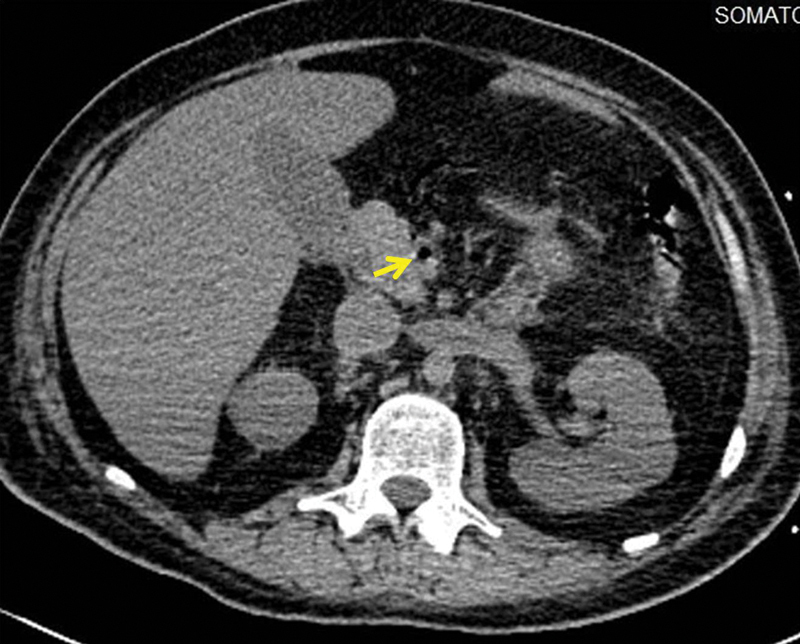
Axial noncontrast computed tomography (CT) scan image showing gas in the superior mesenteric vein (
